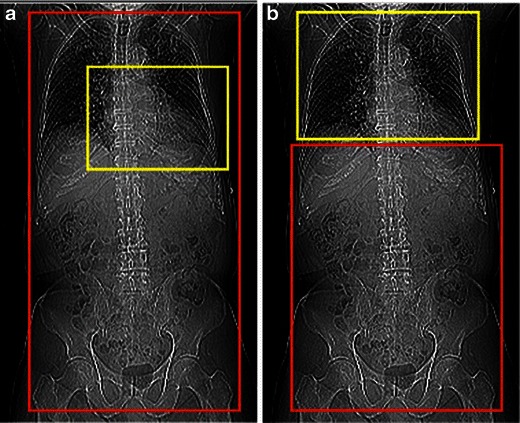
Two types of protocols used based on MDCT scanner parameters: Two scout views corresponding to a 64-slice scanner (a) and 320- slice scanner (b) demonstrate the approach to data acquisition. First, the gated (yellow rectangle) part of the exam is obtained, including only cardiac CTA with the 64-slice scanner and gated CTA of the chest with the 320-slice scanner. The second step (*red rectangle*) corresponds to CTA chest, abdomen and pelvis in the first scenario (a) and CT abdomen and pelvis in the second (b). Note that in both cases eventually the entire torso is imaged
